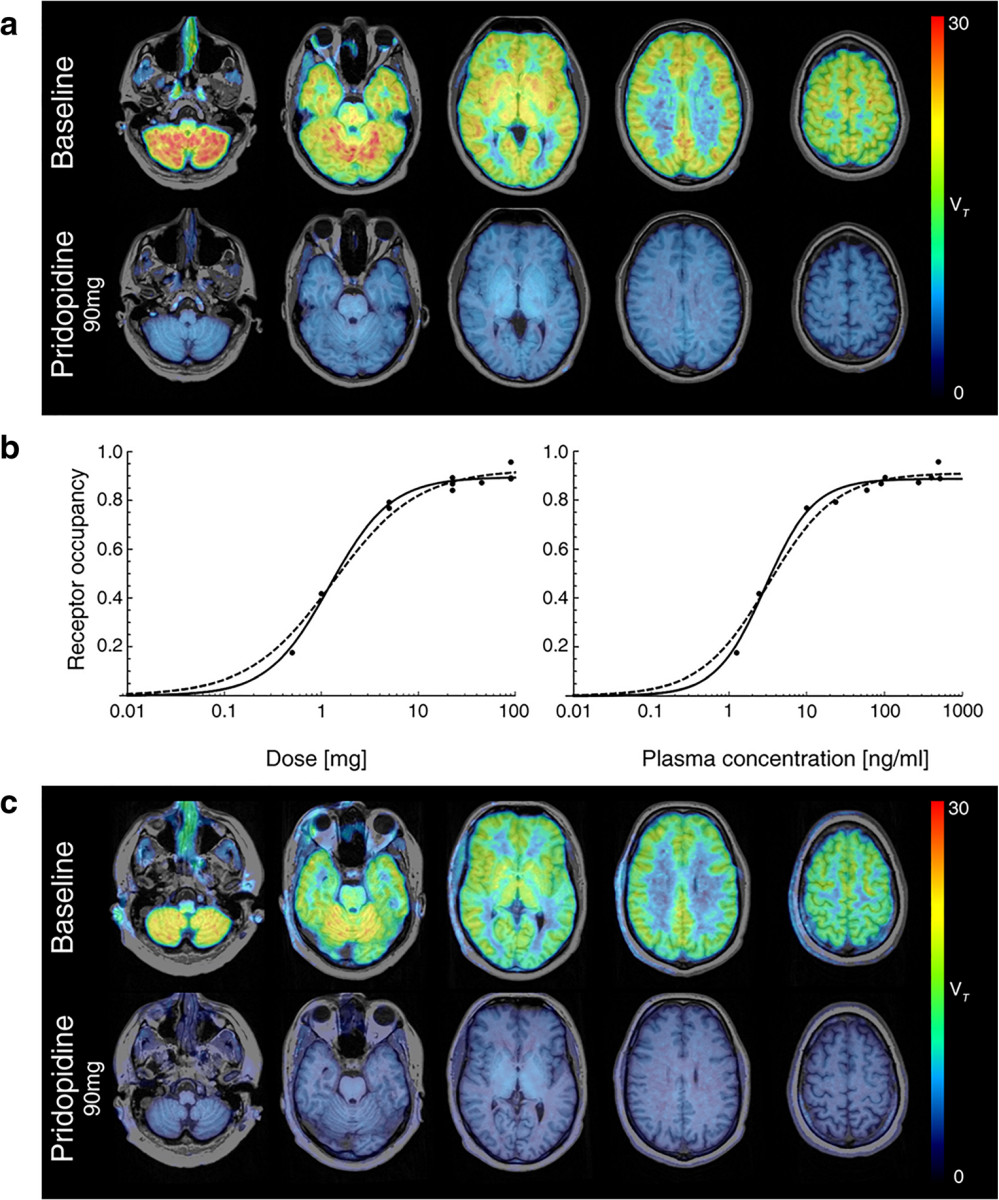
[^18^F] fluspidine baseline and post-drug PET of sigma-1 receptor (S1R) availability in healthy volunteers (HVs) and patients with Huntington disease (HD) and non-linear relationship between pridopidine dose (or plasma concentration) and S1R occupancy. [^18^F] fluspidine PET of S1R availability at baseline and post-drug in HVs and patients with HD. Almost complete S1R engagement/occupancy (*V*_T_) by pridopidine exemplified in one healthy volunteer (a) and one HD patient (c) is demonstrated within the whole brain at post-drug PET (90 mg pridopidine) as compared with baseline PET. For visualization purpose, parametric PET/MR images are shown. There is a sigmoidal curve relationship (b) between the pridopidine dose (left) or plasma concentration (*C*_avg2-4h_; right, both on logarithmic scale) and S1R occupancy in HVs treated by a single dose of pridopidine ranging from 0.5 to 90 mg. The continuous line curve reflects fitting with a three-parameter model (Hill coefficient > 1) which was preferred, whereas the dotted line curve presents a two-parameter type model (Hill coefficient = 1)
